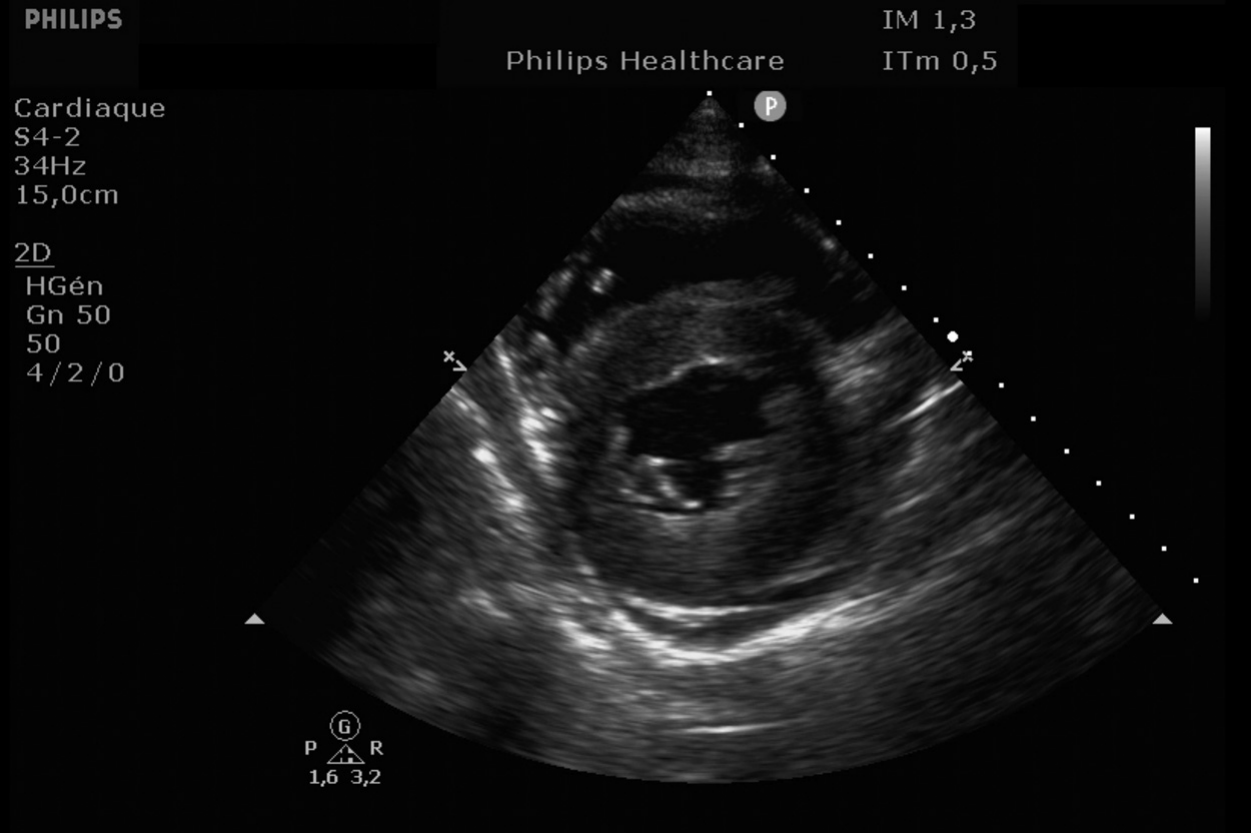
Parasternal short axis window
